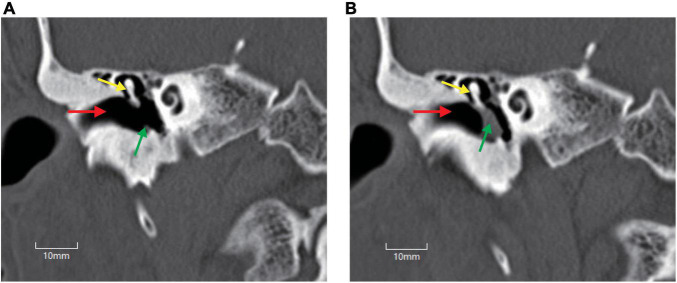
Temporal bone high-resolution CT (HRCT) before and after operation. (A) HRCT before operation. (B) HRCT after operation. Perforation of the tympani membrane (green arrow in A) was repaired using a tragus cartilage (green arrow in B), and the middle ear was reconstructed to a normal anatomical structure to the maximum extent. Red arrow, external auditory canal; yellow arrow, malleus.
